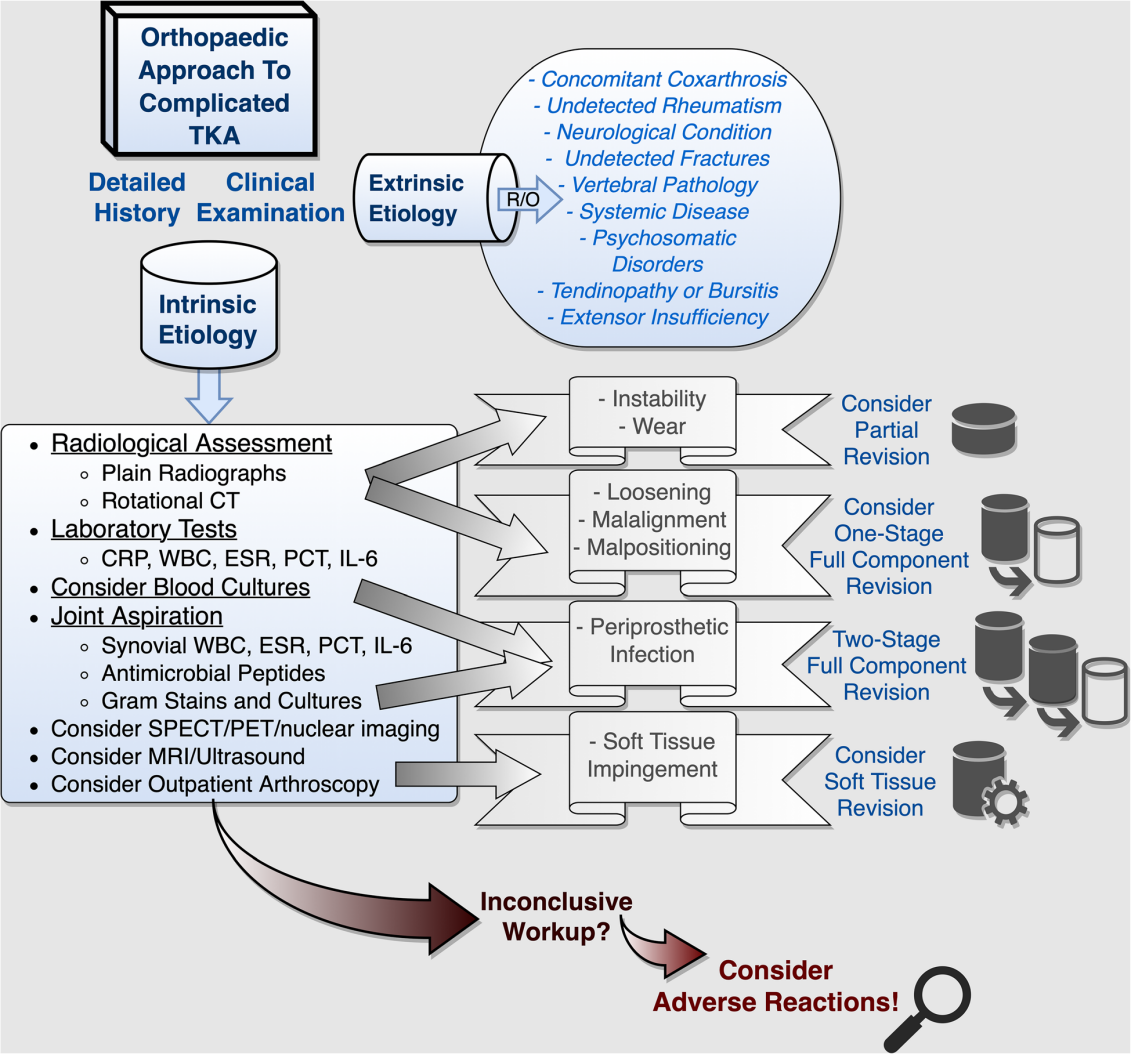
Traditional orthopaedic work-up of common problem elicitors. The initial work-up for complicated TKAs is carried out by the treating orthopaedic surgeon and commences with detailed history taking and a through clinical examination. Once systemic and extra-articular etiologies are ruled out, specific TKA-related problems are addressed. In cases of inconclusive work-up, rare conditions, such as allergic or adverse reactions, should be considered as diagnoses of exclusion (R/O, rule out; CT, computed tomography; CRP, C-reactive protein; WBC, white blood cell count; ESR, erythrocyte sedimentation rate; PCT, procalcitonin; IL-6 , interleukin-6, SPECT, single photon emission computed tomography; PET, positron emission tomography; MRI, magnetic resonance imaging)
